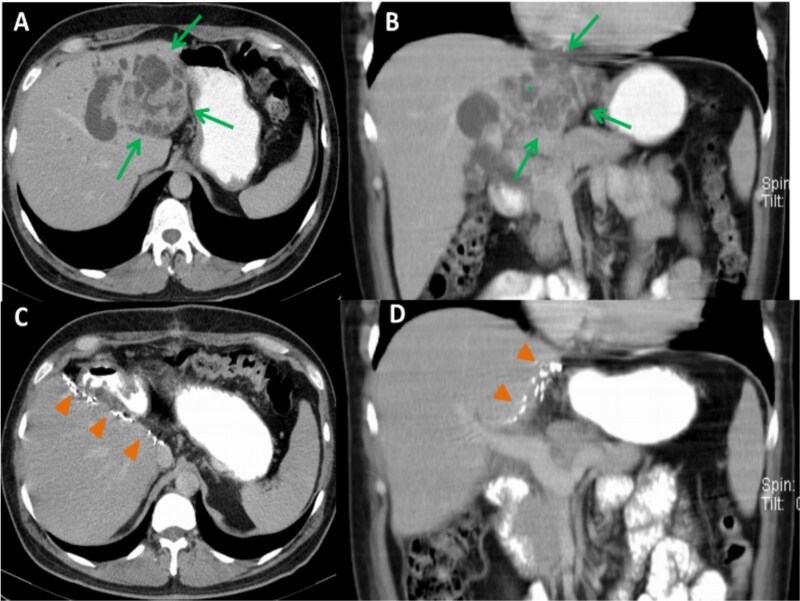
Preoperative CT images, axial (A) and coronal (B) planes, show a large, polycystic lesion in the left hepatic lobe (arrows), dilated intrahepatic bile ducts, and the “central dot” sign (*). Postoperative axial (C) and coronal (D) CT images after left hemihepatectomy show no focal lesion in the right hepatic lobe.
